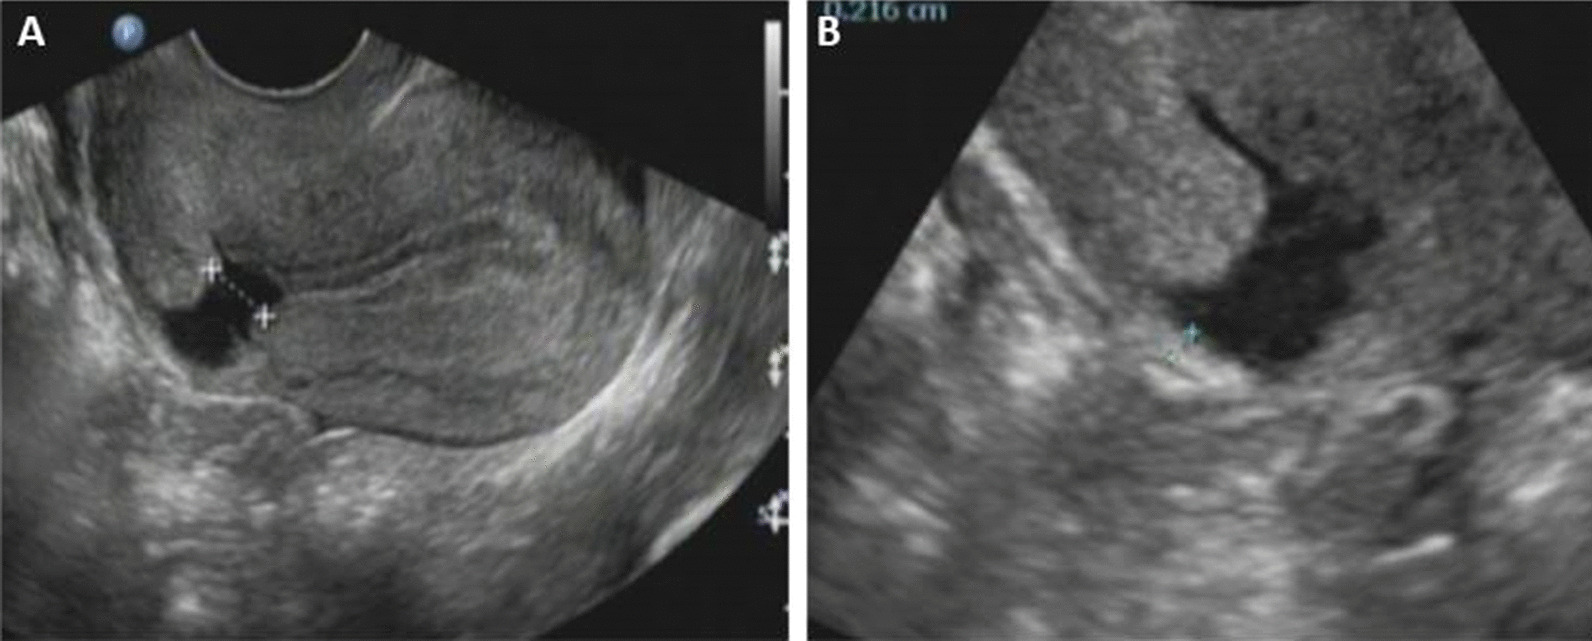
a CSD under transvaginal ultrasound; b TRM under transvaginal ultrasound
